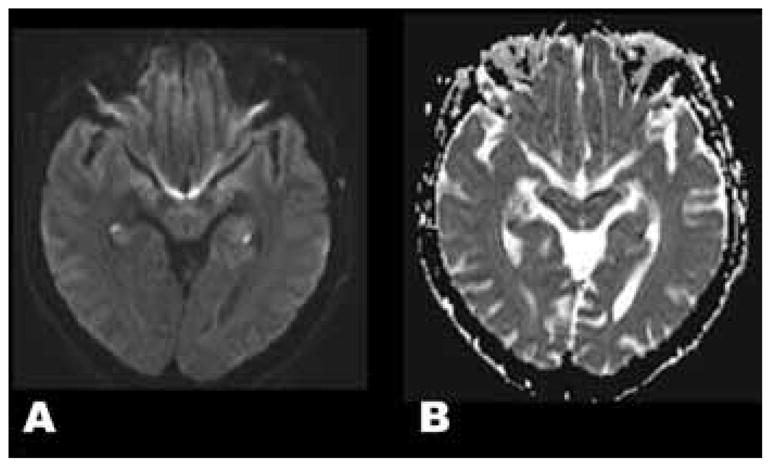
Magnetic resonance imaging scan of the brain (A) Showing bilateral hyperintense posterior portions of the hippocampi, (B) Hypointense apparent diffusion coefficient map in similar brain loci, both compatible with ischemic lesions. Follow-up Magnetic resonance imaging conducted six weeks later showed complete resolution of the lesions.
